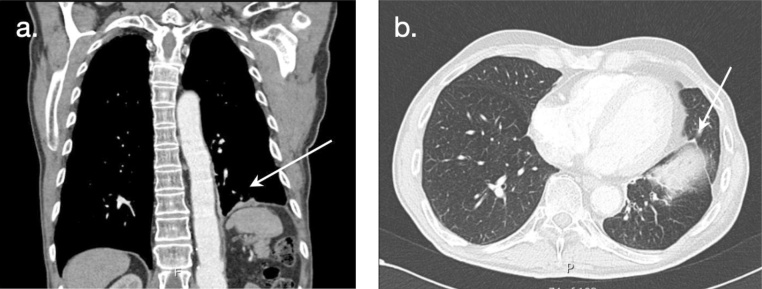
A–B. Post-operative thoracoabdominal computed tomography scan: a satisfactory correction of the diaphragmatic hernia.
